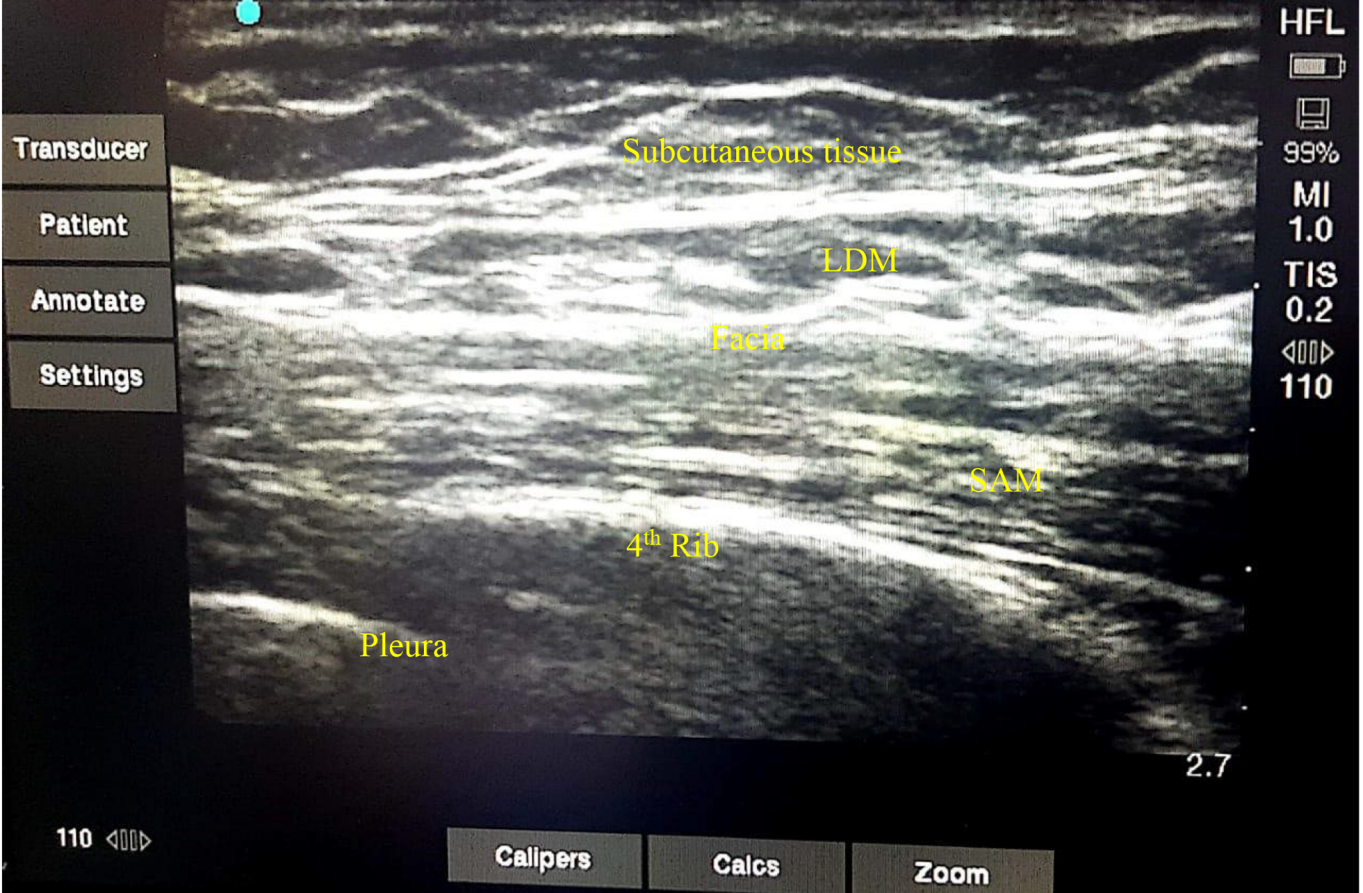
Ultrasound view of serratus anterior plane block at the mid-axillary line. SAM, serratus anterior muscle; LDM, latissimus dorsi muscle.
